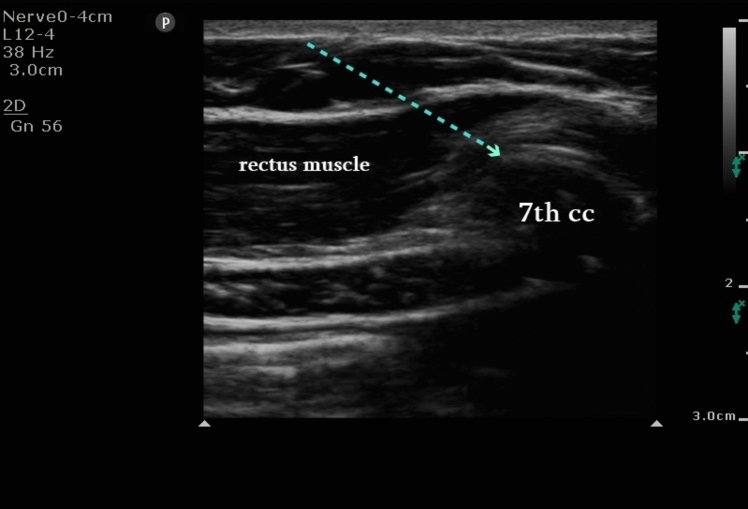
Sonographic visualization of RIFPB. The arrow indicates the needle trajectory, cc costal cartilage
